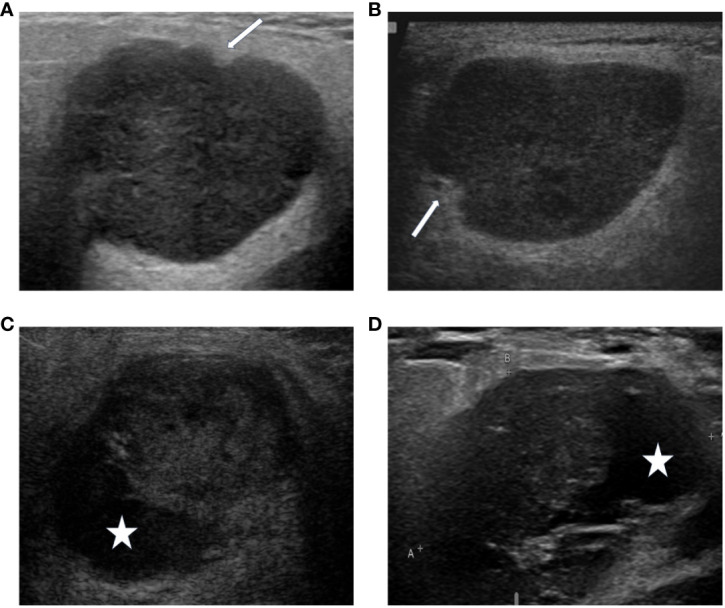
The DL algorithm correctly classified (A) biopsy-PA nodule with a well-known WT appearance, the lobulated borders (A) or the cystic components (C), as PA. The sonographic features demonstrate similarities of the lobulated borders (A, PA), (B, WT), and the cystic components (C, PA), (D, WT). The arrows indicate the lobulated borders of the masses, and the asterisks indicate the cystic components of the masses.
